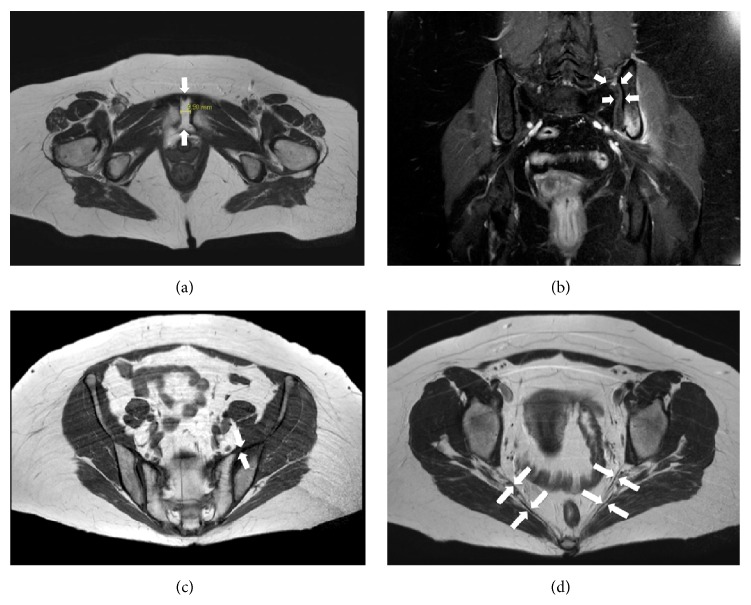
MRI of the pelvis. (a) Axial MRI presenting extensive widening of the symphysis about 9.9 mm (arrows). (b) Coronary MRI also showed widening of the left sacroiliac joint (arrows) and an increased signal from the left ilium. (c) Axial MRI revealed ruptured left anterior sacroiliac ligaments and intact posterior sacroiliac ligaments (arrows). (d) Axial MRI showed intact sacrotuberal and sacrospinal ligaments on both sides (arrows).
